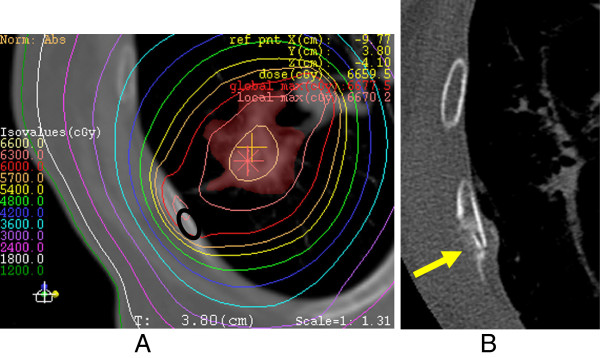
An 86-year-old woman with adenocarcinoma after SRT. A) Dosimetry overlaying CT with a bone window shows the maximum prescribed dose to the chest wall as 63 Gy, with a BED_3_ of 233.2 Gy. The site of rib fracture later is indicated by a black elliptical cercle. B) Rib fracture was noted at 24 months after completion of SRT. Amorphous osteosclerosis is also seen (arrow).
